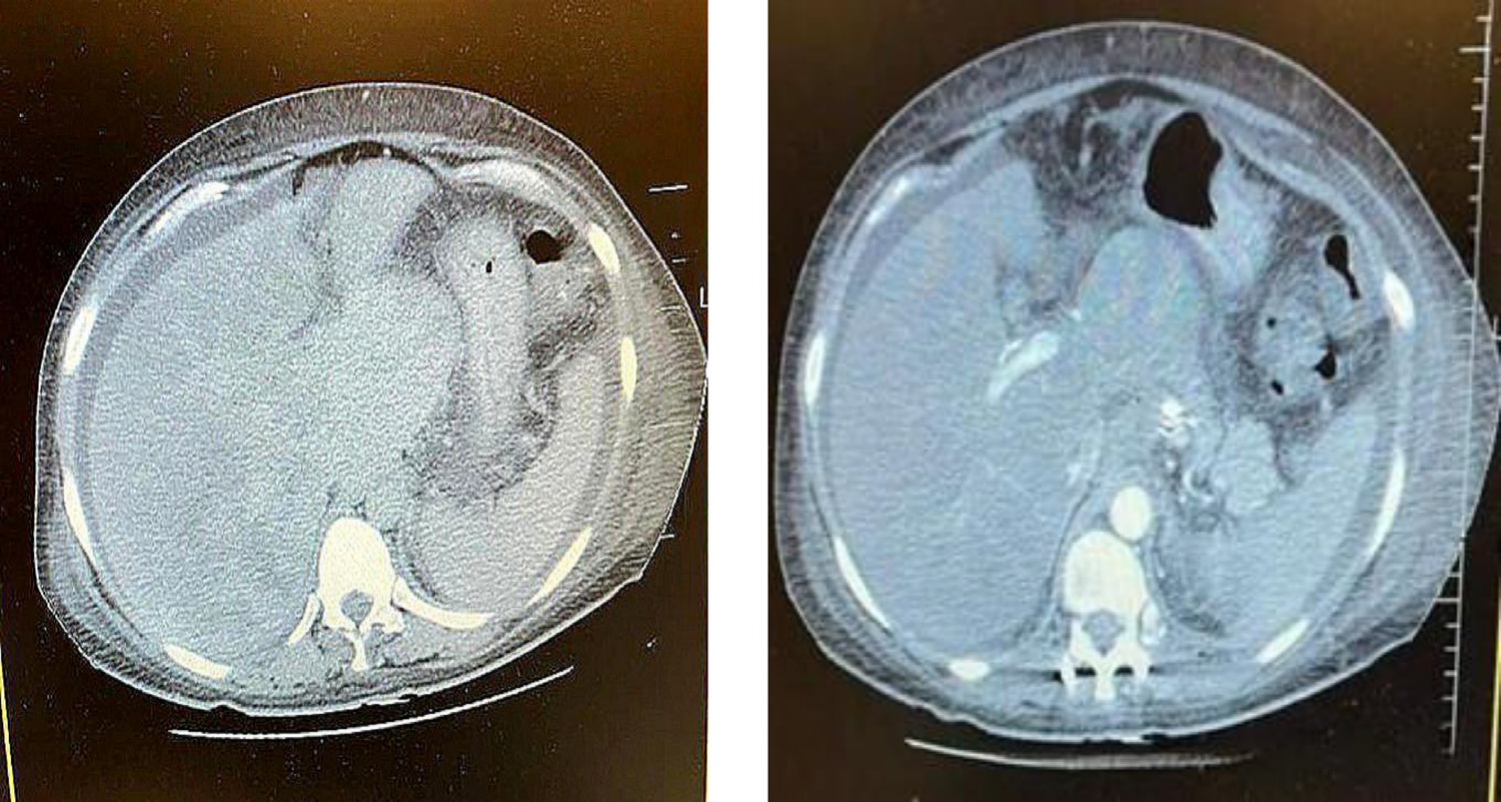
Abdominal CT scan: Hepatomegaly with heterogeneous enhancement. Narrowed or indistinct hepatic veins, supporting Budd-Chiari syndrome. Significant ascites and mesenteric venous congestion. Mild splenomegaly, suggesting portal hypertension.
